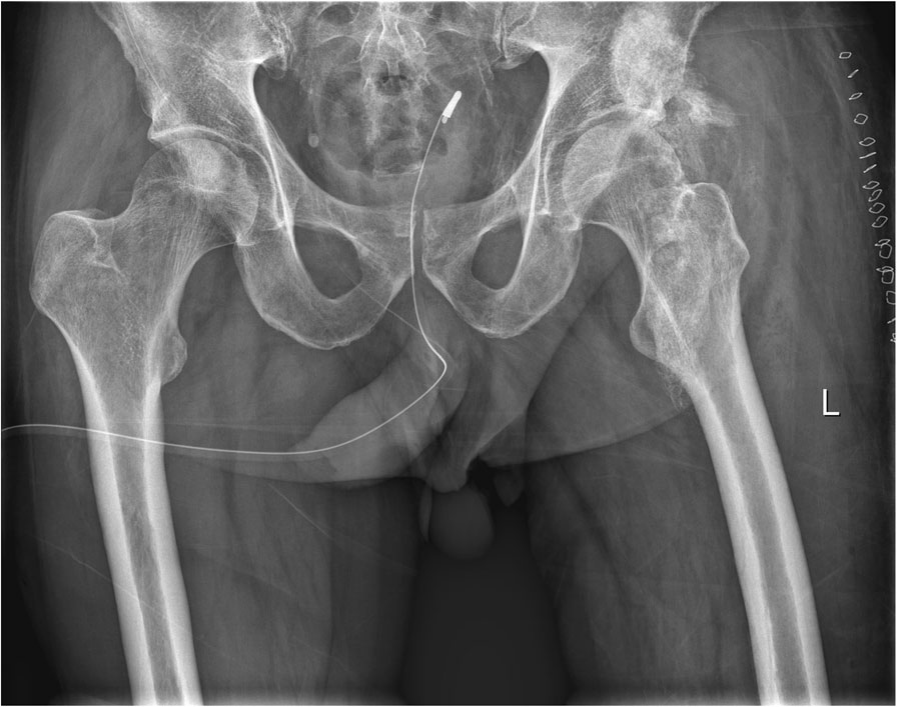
Radiograph of the HTO of the left hip
